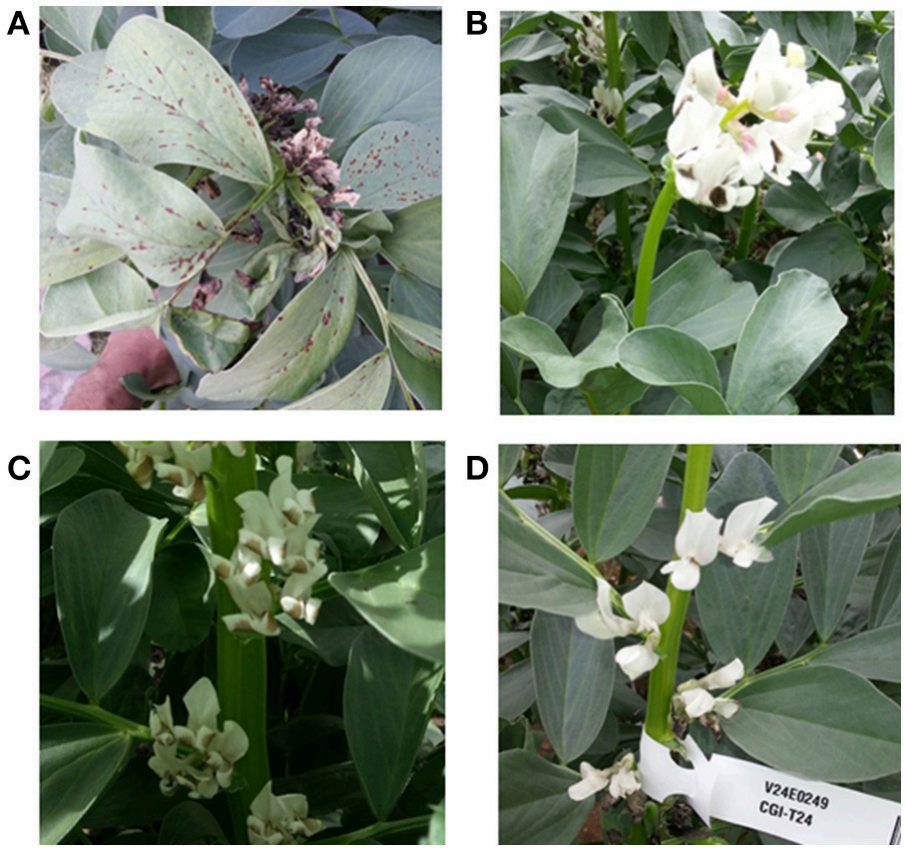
Mutant phenotypes observed in X-ray and EMS M_2_ lines grown at the University of Reading in 2015. (A) disease lesion mimic, (B) determinate flowering, (B) curled wing petal, (C) disease lesion mimic, (D) reduced flower pigmentation.
